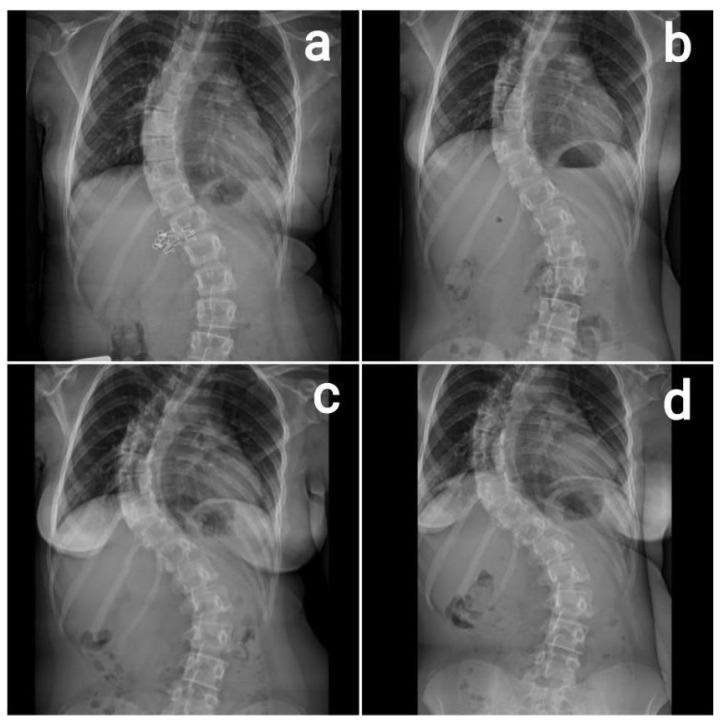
(a) First examination: 52 degrees by Cobb. Lenke 3C. (b) Three months after the first examination: 58 degrees by Cobb. Lenke 3C. (c) Nine months since first examination: 78 degrees by Cobb. Lenke 3C. (d) Last preoperative X-ray.
